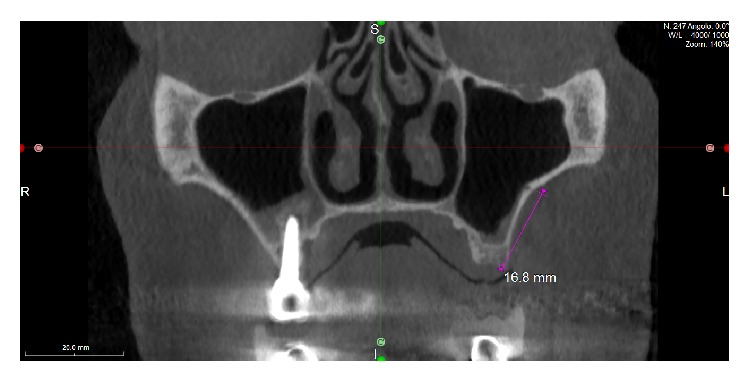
CBCT of the sinuses. The maxillary intraosseous anastomosis at the left sinus is 16.8 mm above the ridge coronal bone level.
